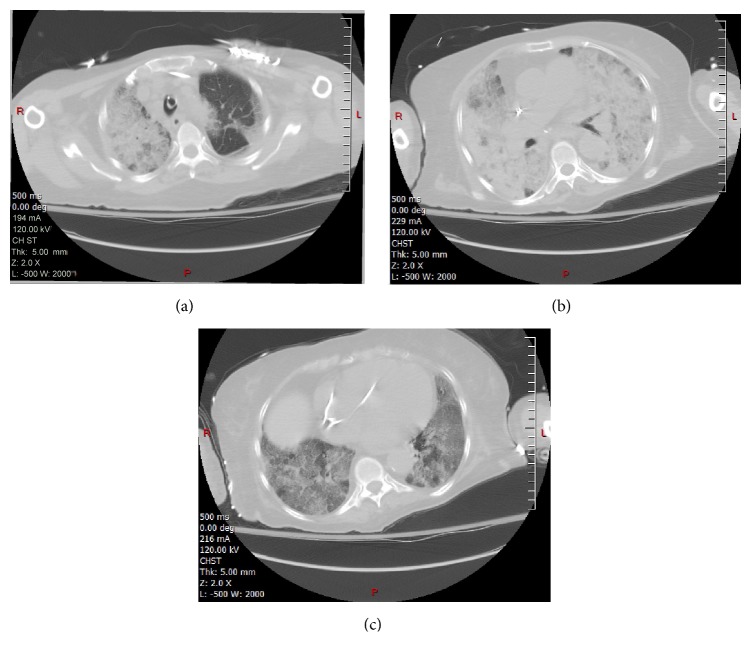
CT scan images above the plane, thru the plane, and below the plane of SBRT treatment showing diffuse infiltrates ((a), (b), and (c) images respectively).
